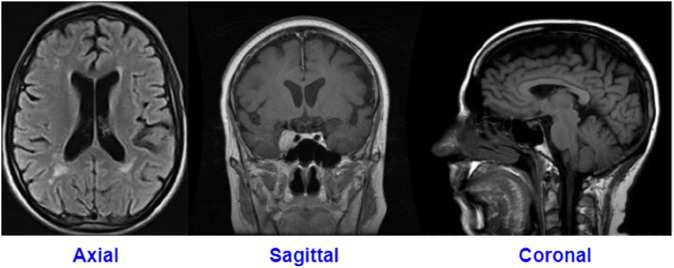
MRI image planes (this MRI sample from dataset A and dataset B).
